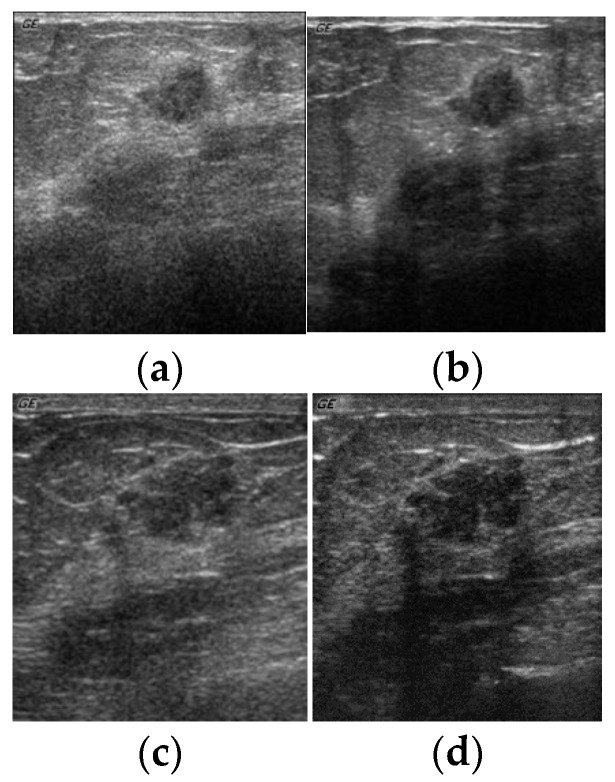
Ultrasound breast cyst image for four distinct settings of the parameters acquired from the same ultrasound sensing system (GELOGIQ 700). Breast cyst images acquired from (a) Parameter Setting-1, (b) Parameter Setting-2, (c) Parameter Setting-3, and (d) Parameter Setting-4.
